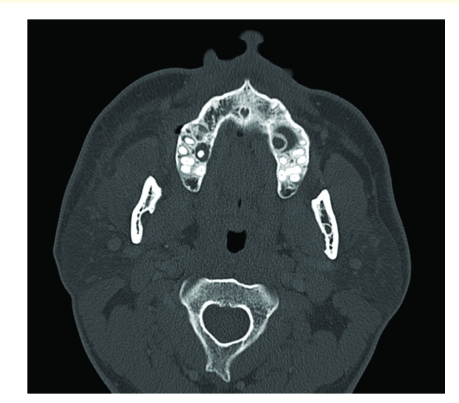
Axial CT scan (admission) shows bilateral periapical dental granulomas
